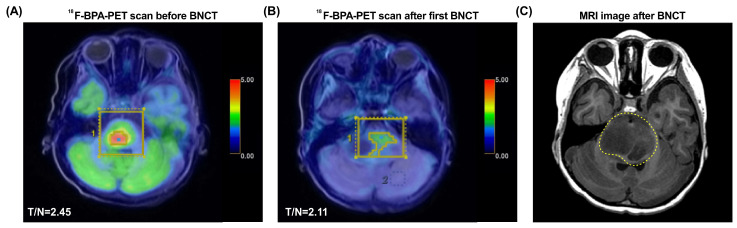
^18^F-BPA-PET and MRI images of Case 1 (patient #5). (A) The ^18^F-BPA-PET reveals the location of tumor activity before BNCT treatment. (B) The ^18^F-BPA-PET reveals the location of tumor with reduced tumor activity after first procedure of BNCT treatment. (C) The follow-up MRI image of the patient shows radiation necrosis in the fourth ventricle (dashed yellow line). The yellow squares indicate the tumor location before and after BNCT treatment.
